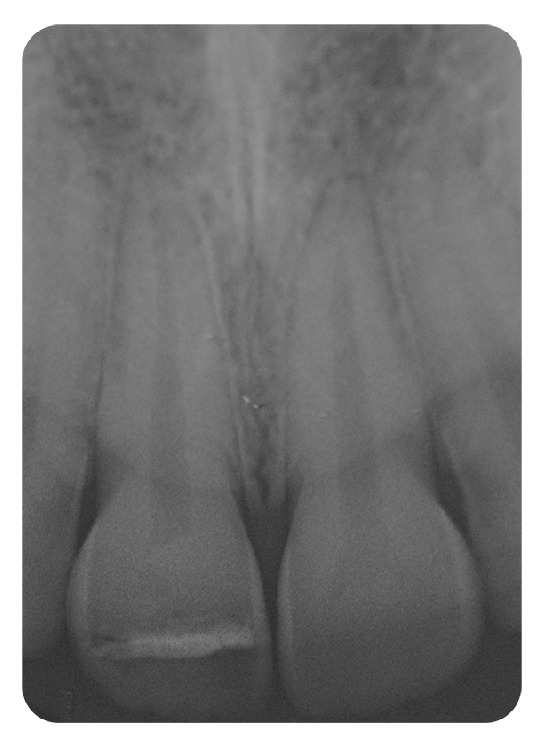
Radiograph showing periapical radiolucency.
